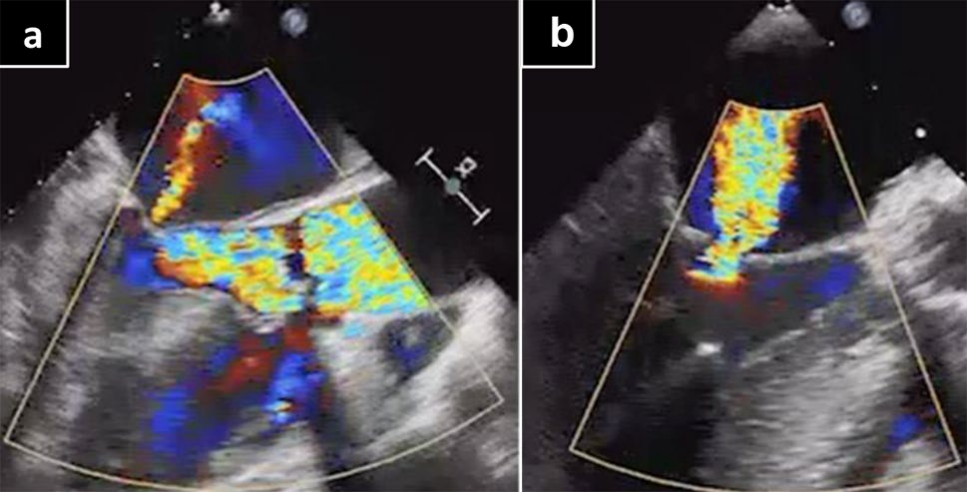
a, b. Mitral valve regargitation with central jet. (a) Preoperative and (b) intraoperative echocardiography
